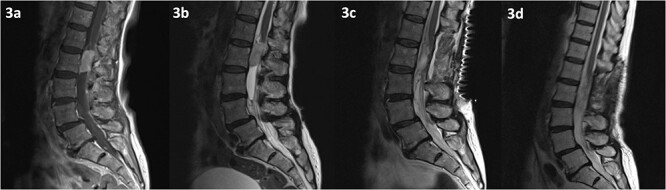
MRI of the spine depicting an intradural lesion at the level of L2, just below the conus. The lesion demonstrates homogenous enhancement on contrast enhanced T1-weighted imaging (a). The lesion is hyperintense on T2-weighted imaging (b). There is no evidence of residual tumour on T2 weighted imaging at 2 days (c) and 14 months (d) postoperatively.
